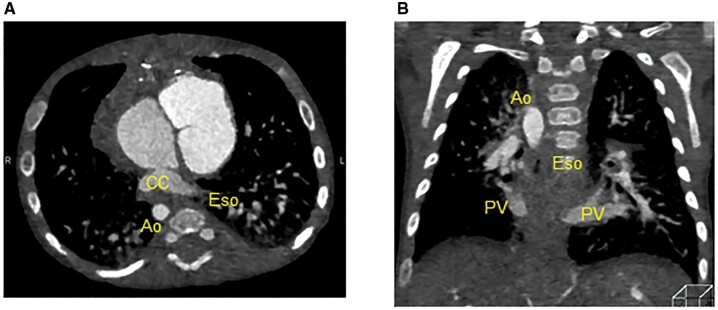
The complete regression of the oesophageal varices in postoperative computed tomography. (A) The axial image, (B) the coronal image of postoperative computed tomography. Ao: aorta; CC: common chamber; Eso: oesophagus; PV: pulmonary vein.
